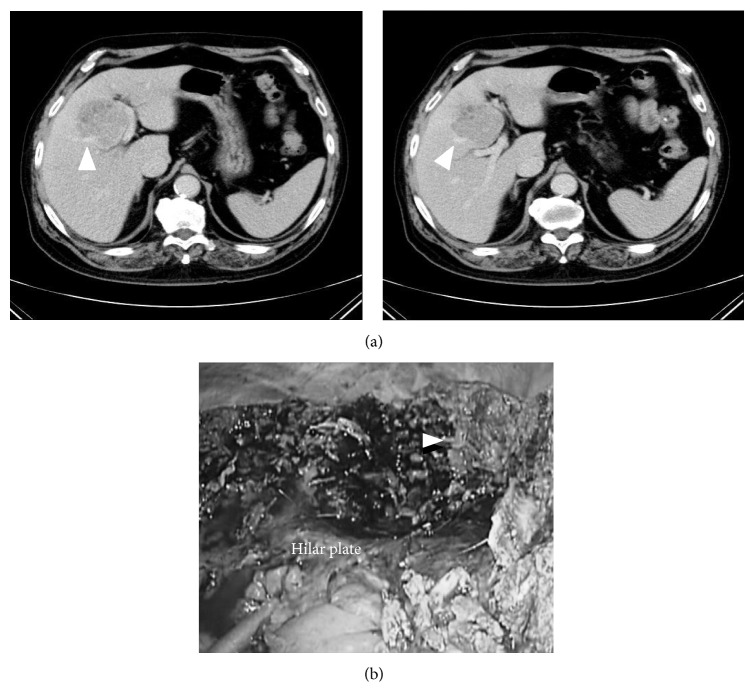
Preoperative CT findings (a) and intraoperative findings after completion of the resection (b), case 2 (left medial sectionectomies combined with middle hepatic vein resection). 70-year-old man with type-C chronic hepatitis developed 7.5 cm HCC in segment 4–8. (a) The tumor compressed the Glissonian pedicle widely, from hilar plate to umbilical plate and to anterior branch, and also there was an involvement of the middle hepatic vein (arrow head). This patient underwent extended left medial sectionectomy combined with the middle hepatic vein resection. (b) After completion of liver resection, the Glissonian pedicle, from hilar plate to umbilical plate and to anterior branch, was exposed and the stump of middle hepatic vein (arrow head) was clearly shown in the operative field.
